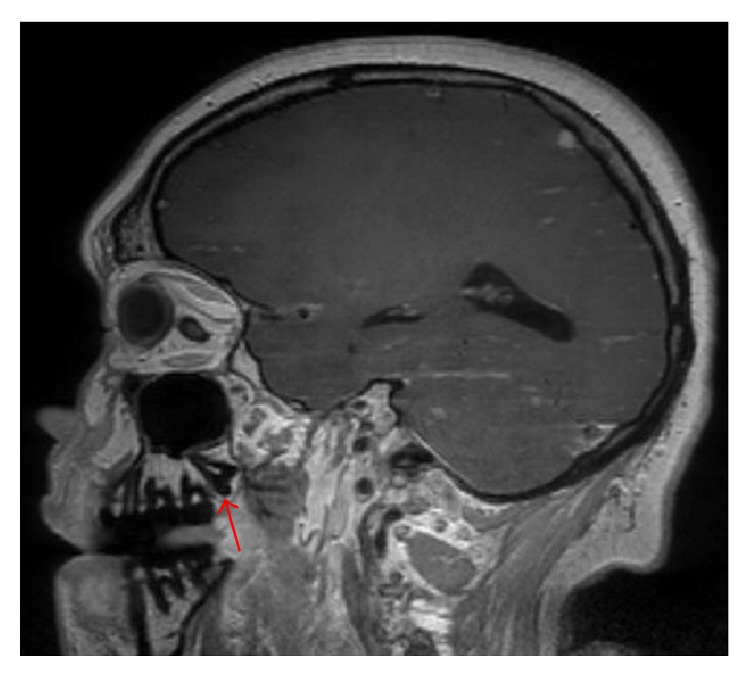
Impacted upper right third molar seen on MRI in close proximity to diseased pituitary gland.
